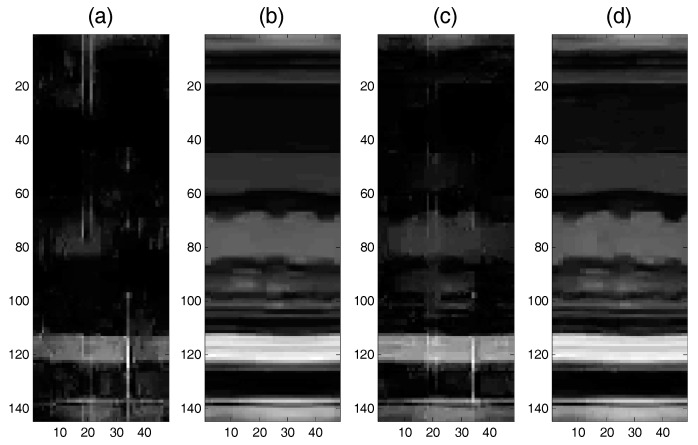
The recovery effects of the proposed SAITA-$L_{p}\left(p\in \left\{1/2,\;2/3\right\}\right)$ and the corresponding $L_{1/2}$ and $L_{2/3}$ algorithm for the M/N=0.2, mSNR=40 dB 2D MRI image. (a)$\;L_{1/2}$ algorithm, RSNR=3.6346 dB; (b) SAITA-$L_{1/2}$ algorithm, RSNR=21.0189 dB; (c) $L_{2/3}$ algorithm, RSNR=7.7718 dB; and (d) SAITA-$L_{2/3}$ algorithm, RSNR=23.1872 dB.
